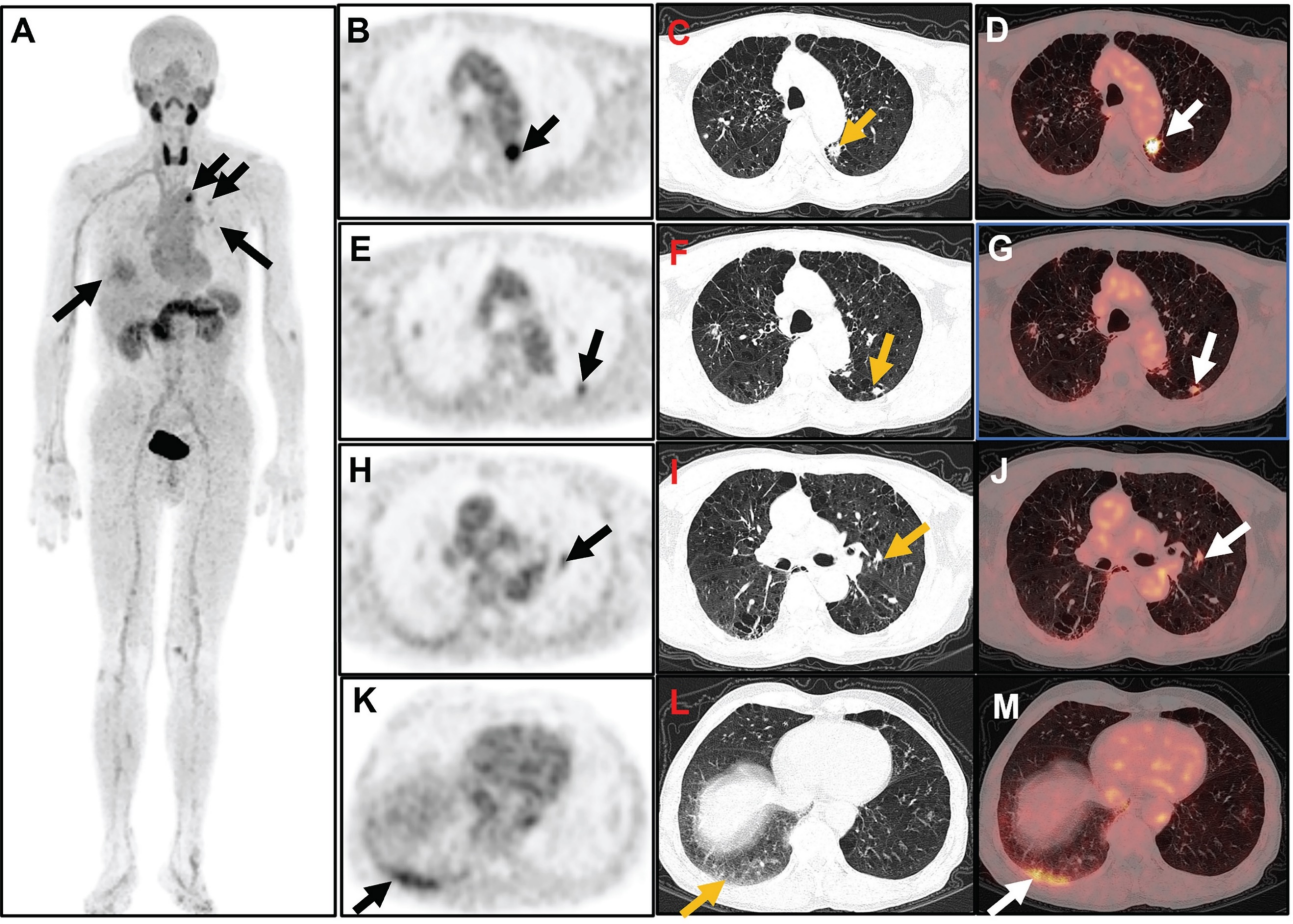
[^18^F]AlF-FAPI-RGD-PET images of a 59-year-old man (Participant 17) with a 38-month history of RA and ongoing disease activity. The participant has a DAS28-ESR score of 6.1, DAS28-CRP score of 5.5, CDAI of 31.0, and SDAI of 45.2. Whole-body MIP image (A) shows various degrees of increased uptake of FAPI-RGD at multiple sites in the bilateral chest. Axial PET/CT images revealed increased FAPI-RGD uptake in a small patch in the upper left lung (arrow; B, PET; C, CT; D, fusion; SUV_max_ 8.1), a small nodule in the dorsal segment of the lower lobe of the left lung (arrow; E, PET; F, CT; G, fusion; SUV_max_ 3.6), a small nodule near the left hilar (arrow; H, PET; I, CT; J, fusion; SUV_max_ 3.3), and a ground-glass density patchy shadow at the base of the right lung (arrow; K, PET; L, CT; M, fusion; SUV_max_ 4.3).
